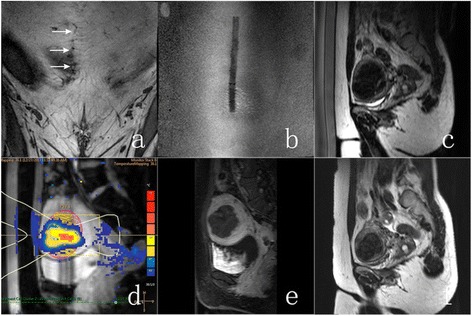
A 43-year-old woman who had a longitudinal incision presented with menorrhagia was treated with MRI-guided high-intensity focused ultrasound ablation using scar patch. a Scar scan showing the orientation of the scar within the abdominal fat layer (scar location identified with *white arrows*). b Scar scan showing the air-containing scar patch at the patient’s skin. c T2w planning image acquired prior to the therapy. d PRF thermometry scan acquired during one of the sonications. e T1w gadolinium-enhanced image acquired immediately after the therapy showing the induced necrosis. f T2w planning image at 6-month follow-up
